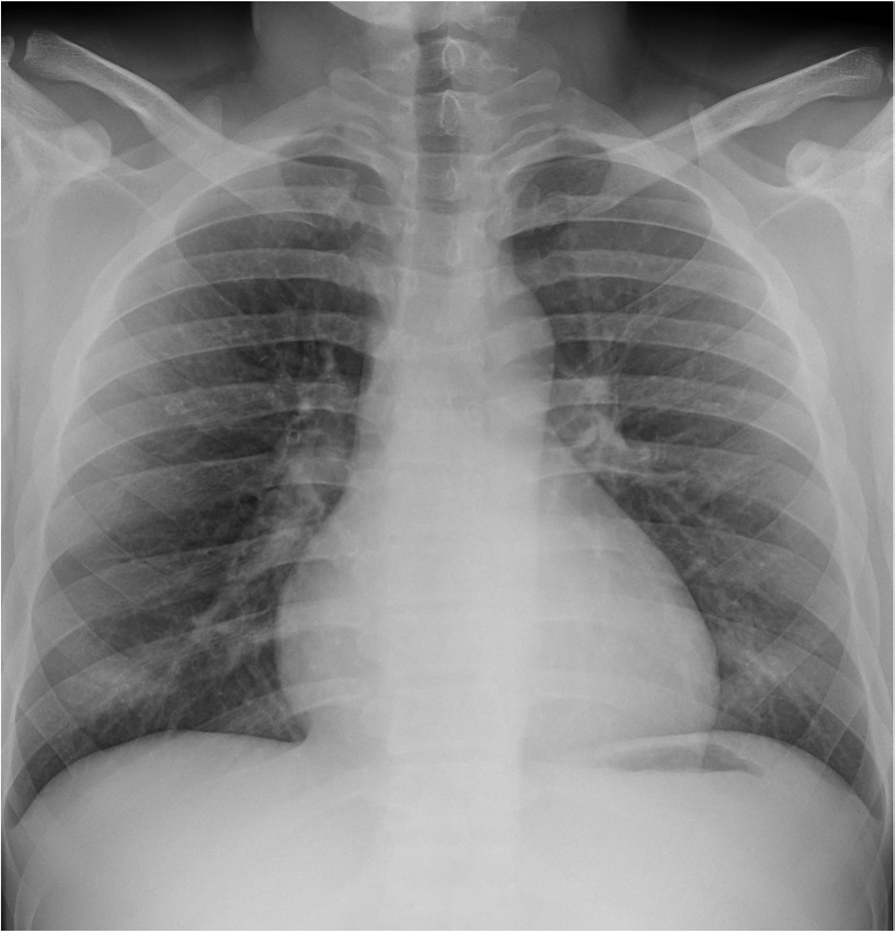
A 17-year-old boy with COVID-19 pneumonia who presented with an upper respiratory tract infection. The chest radiograph shows bilateral ground-glass opacities in both lower lung zones. The findings are consistent with the typical multifocal pattern of COVID-19 pneumonia. The severity scoring in this patient was calculated as the sum of the right lung scores and the left lung scores (total scores = 2 + 2 = 4). COVID-19, coronavirus disease-19
